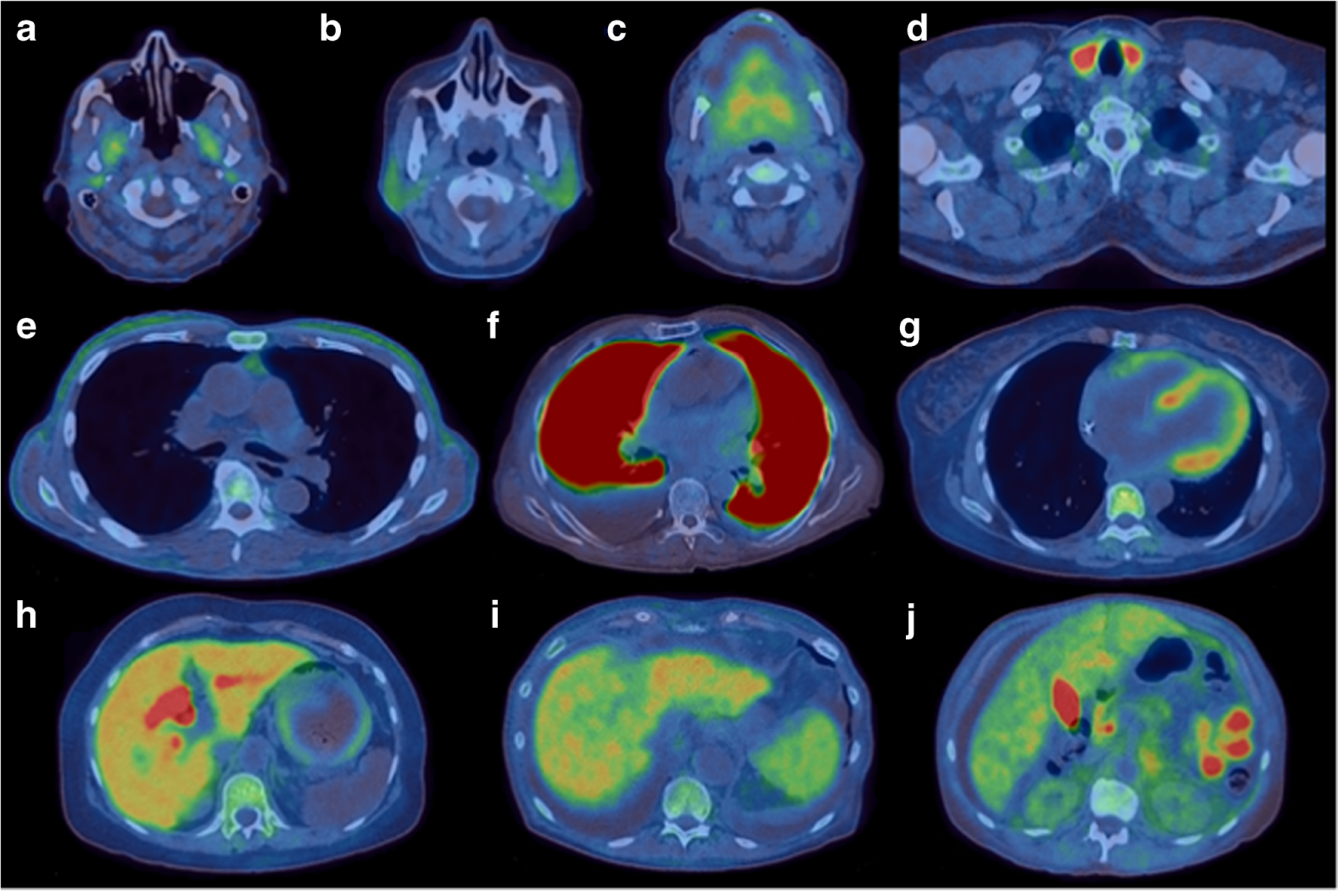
Transaxial ^18^F-florbetapir fused PET/CT images showing representative areas of tracer uptake: a pterygoids (patient 3), b parotids (patient 2), c tongue (patient 17), d thyroid (patient 14), e subcutaneous and pericardial fat (patient 8), f lungs (patient 7), g heart and bone marrow (patient 2), h liver, bile ducts and stomach wall (patient 2), I liver and spleen (patient 1), j liver, gallbladder, small bowel and kidneys (patient 13)
